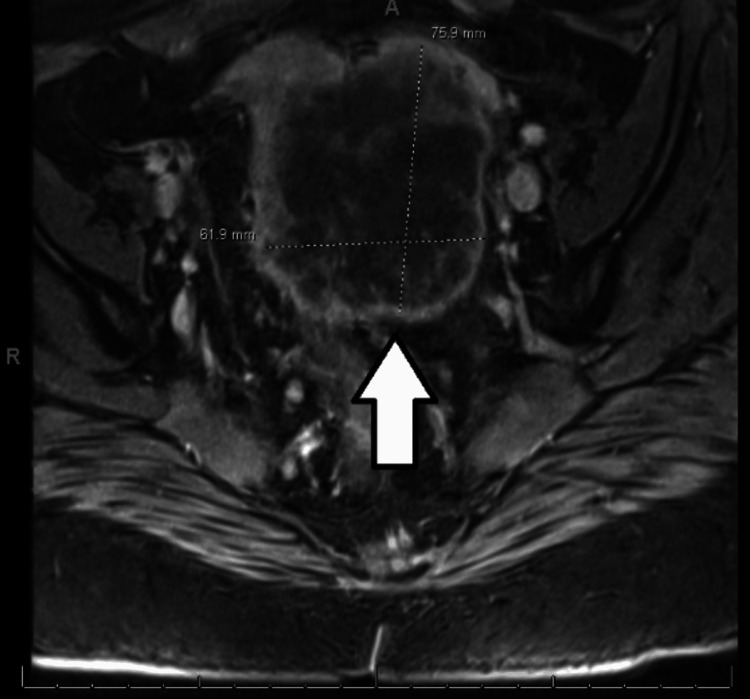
MRI of the pelvis without contrast demonstrating a large mass measuring 7.6 × 6.2 × 6.2 cm present in the pelvis predominantly to the left of the midline
